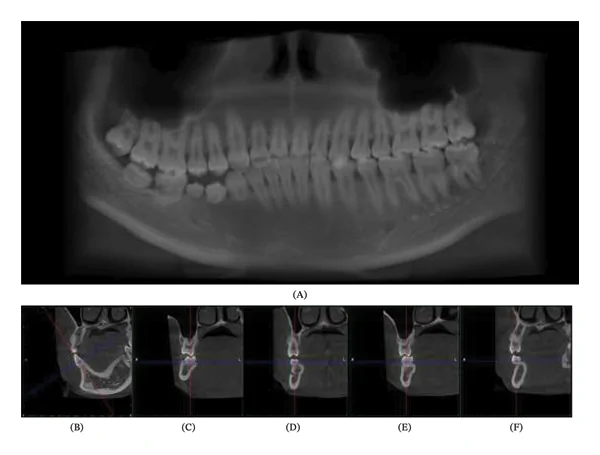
Cone‐beam CT examination. (A) CT cross section showing the development of whole dentition; (B)coronal surface of the right lower cusp; (C) coronal surface of the first right premolar; (D) coronal surface of the second right premolar; (E) coronal surface of the first right molar; and (F) coronal surface of the second right molar.
